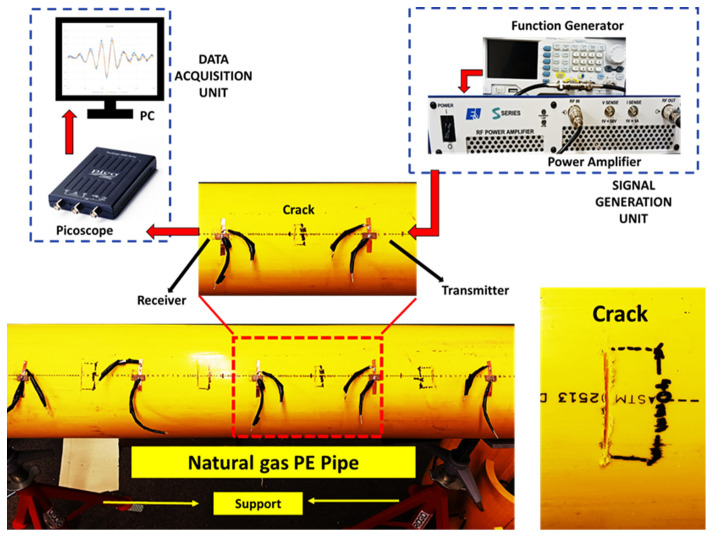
Experimental setup for ultrasonic testing of the PE pipe with crack.
